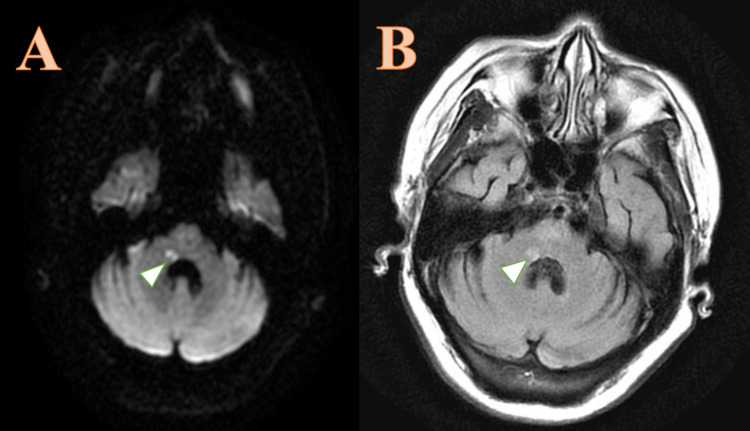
A) Axial diffusion-weighted imaging shows a tiny focus of diffusion restriction in the right pons on the floor of the fourth ventricle in the region of facial colliculus, shown by the arrowhead. (B) Axial T2 fluid-attenuated inversion recovery image (FLAIR) reveals a corresponding focus of hyperintensity signal
